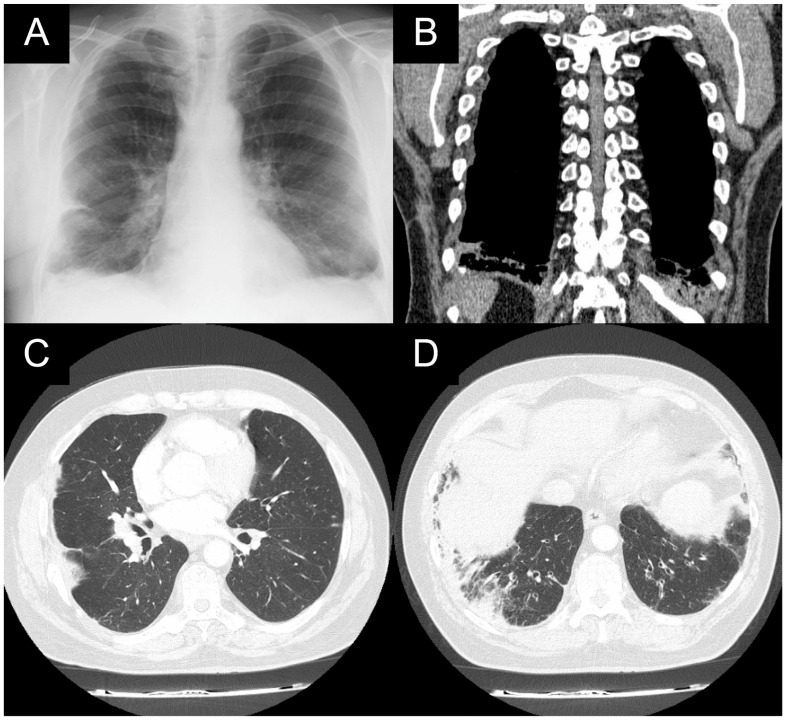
X-ray of the coronal plane (A) from 2017; CT scan: coronal plane (B) and axial plane (C,D) from 2017. Pulmonary infiltrates and consolidations in the radiological images.
